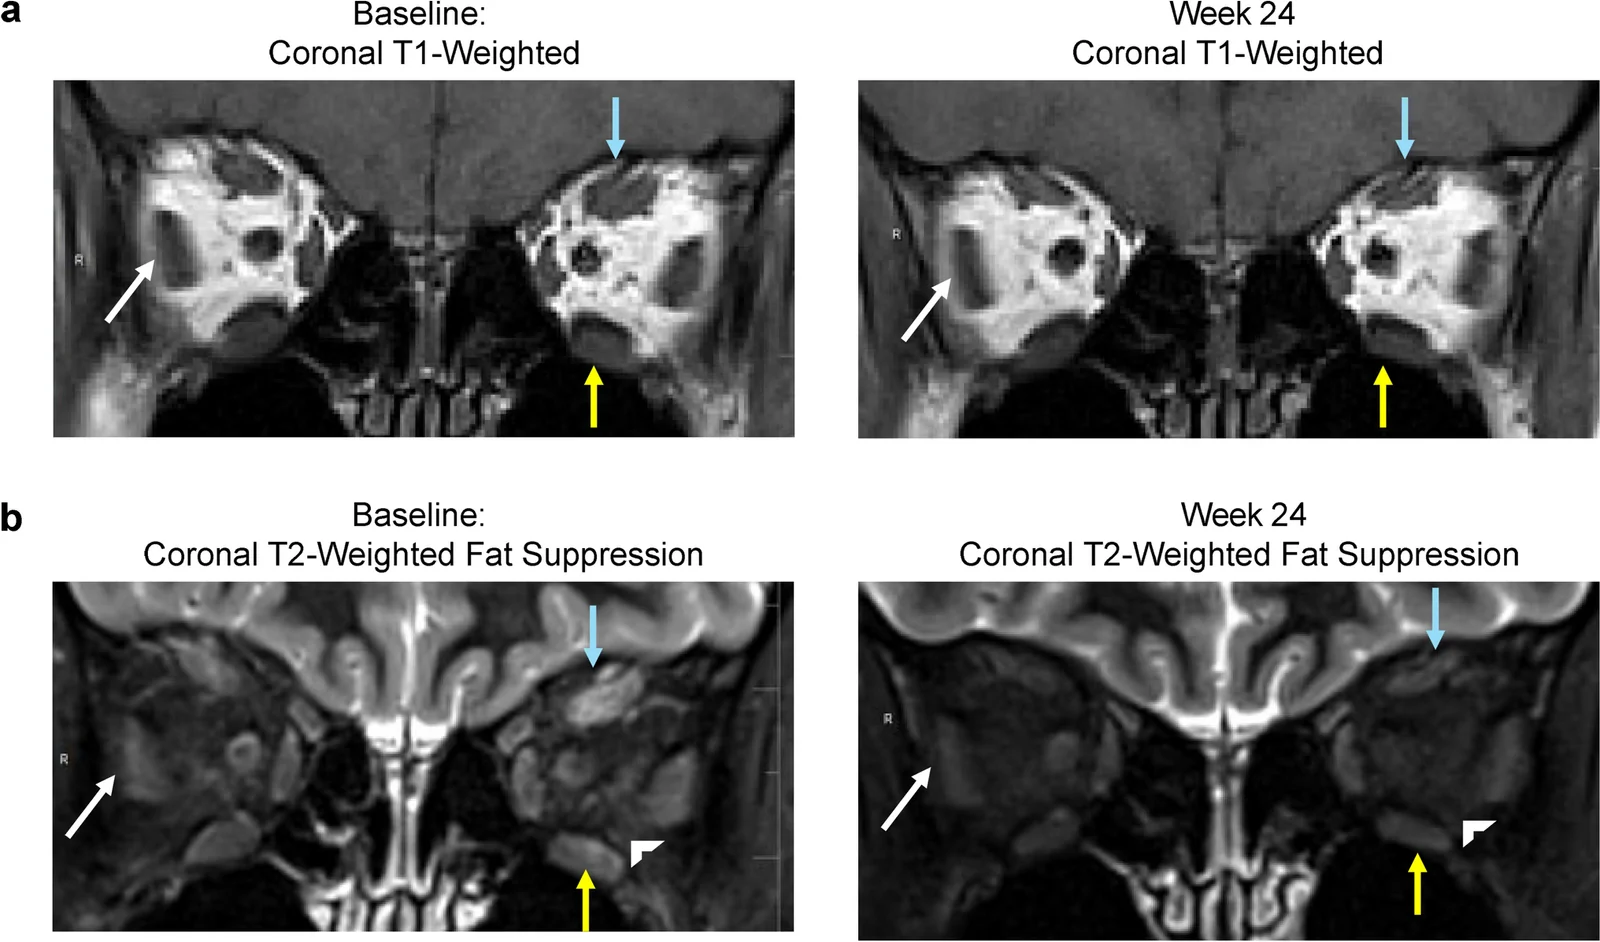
MRI scans taken at baseline and week 24 in a patient treated with teprotumumab demonstrating a reduction in the volume of orbital fat and EOMs with coronal T1-weighted MRI and b reduction in inflammation/edema of orbital fat and EOMs with T2-weighted fat-suppressed scans. Blue arrows indicate the superior rectus; yellow arrows indicate the inferior rectus; white arrows indicate the lateral rectus; and the white arrowhead indicates the orbital fat inflammation. *EOM* extraocular muscle, *MRI* magnetic resonance imaging
